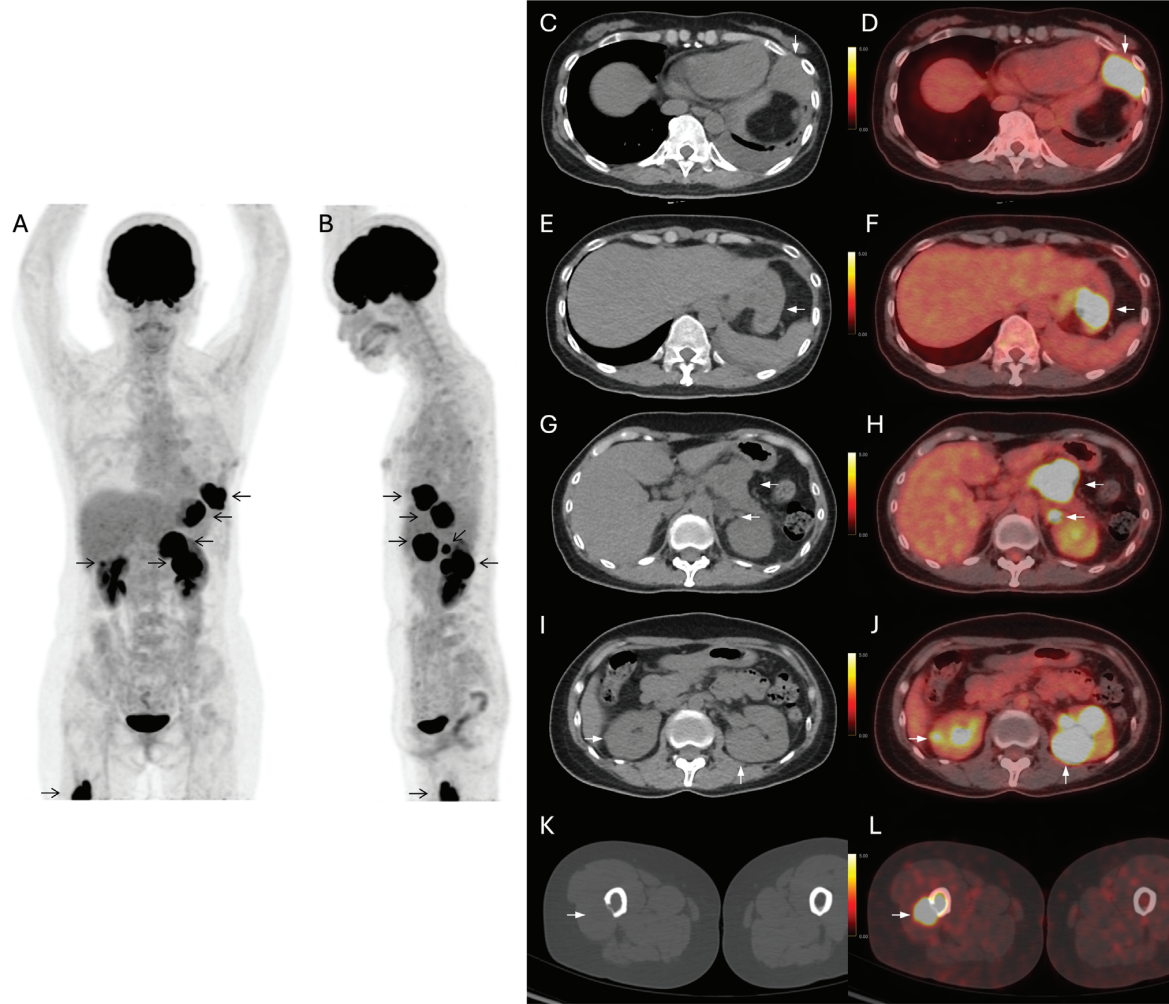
2-deoxy-2-[18F]fluoro-D-glucose positron emission tomography/computed tomography (FDG PET/CT) performed 7 months after lobectomy of left upper lobe of lung for surveillance. (
